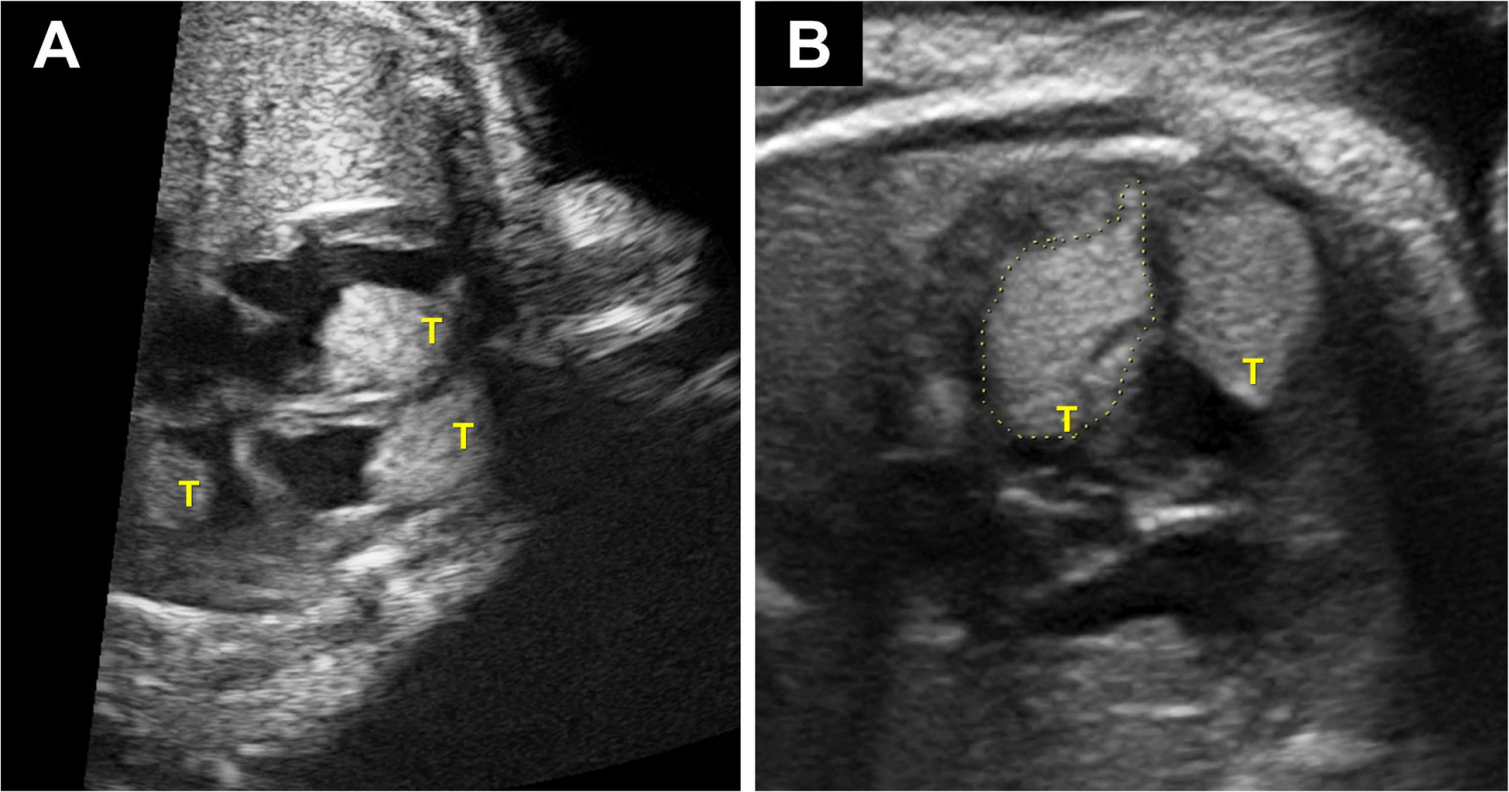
Fetal cardiac ultrasound showing the presence of tumors. a Image showing the two atriums and two ventricles with the presence of tumors (T) in the right atrium (observable on the left), the right ventricle as well as in the left ventricle (observables on the right). b Image showing tumors (T) observed in the left and right ventricles. The largest tumor (6.26 cm circumference), which was observed in the subaortic region of the left ventricle and was slightly obstructive, is surrounded by a dotted line
